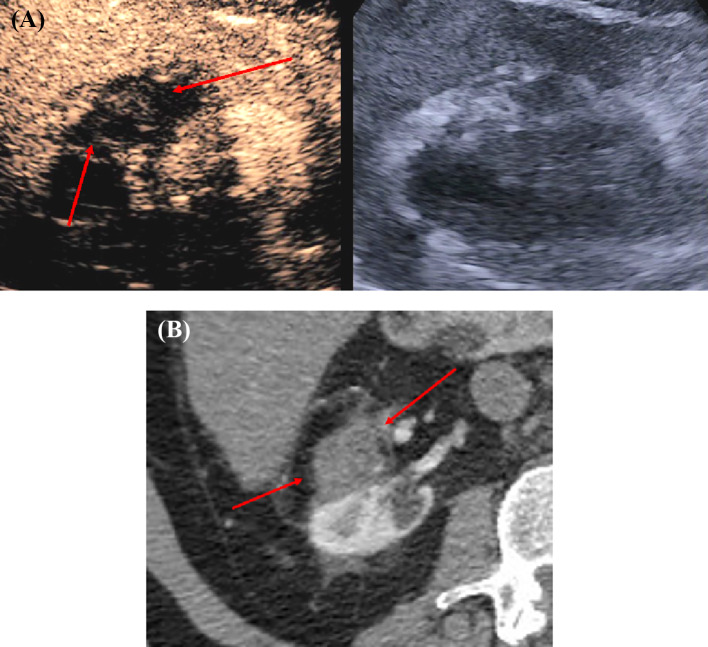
60-year-old male with residual tumor post RFA for a clear cell carcinoma G2 pT1a. one month post ablation. The CEUS (A) is positive with contrast within the ablation site (arrows) while the CECT (B) does not show any enhancement in the ablation site and is negative. The CEUS is a dual screen image with the contrast enhanced ultrasound on the left and a synthesized B-mode image on the right. The B-mode image is used to confirm localization and is not a diagnostic image. The patient is on surveillance monitoring and the enhancement on CEUS has not changed over a 1-year period
